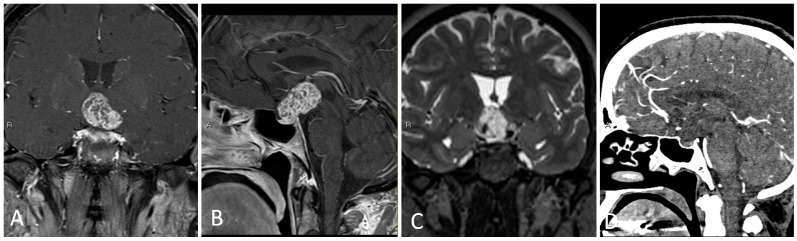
Preoperative imaging of a 56-year-old patient with an enhancing, partially calcified sellar and suprasellar lesion consistent with craniopharyngioma. (A) Coronal T1 post-contrast MRI. (B) Sagittal T1 post-contrast MRI. (C) Coronal T2-weighted MRI. (D) Sagittal thin cut CTA head demonstrating partial peripheral calcification.
